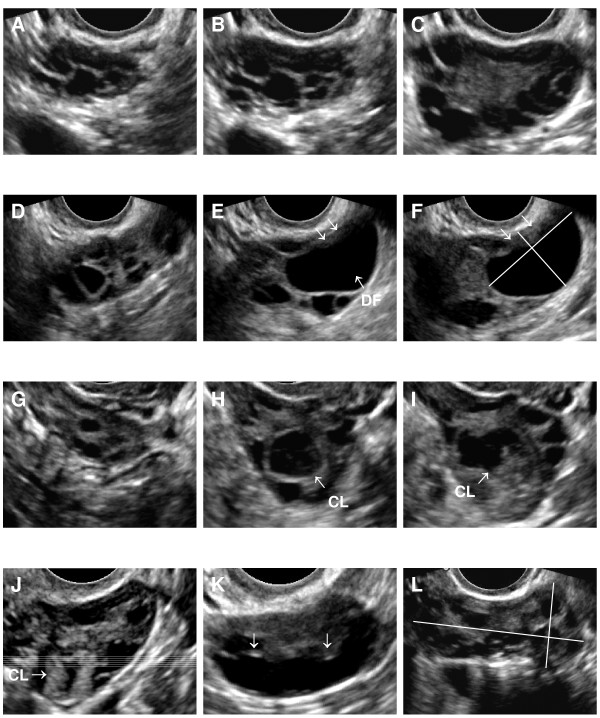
Ultrasonographic image attributes encountered during the inter-observer evaluation of polycystic ovaries are presented. Panels A, B and C represent sequential images through a polycystic ovary where an apparently 'even' distribution of follicles is evident at the end of the ovary while a peripheral distribution of follicles is evident at the central portion of the ovary (Panel C). Designation of follicle pattern was to be made at the largest cross-sectional image of the ovary. Panels D, E and F depict sequential images through a polycystic ovary containing a large dominant follicle (DF). An area of acoustic artifact (arrows) is present along the top portion of the dominant follicle closest to the transducer (Panels E and F). Both the follicle wall and area of acoustic artifact were to be included in the measurements of the largest follicle diameter (Panel F). Panels G, H and I demonstrate sequential images through a polycystic ovary containing a large cystic corpus luteum (CL). The cystic CL has a hyperechoic crenulated border and a flocculent fluid filled cavity. A polycystic ovary containing a CL with thick hyperechoic walls and a small fluid-filled cavity is presented in Panel J for comparison with Panel I. A polycystic ovary containing a concentrated collection of follicles along the lower margin of the ovary is presented in Panel K. The number of follicles counted would depend on the number of lobulations perceived among the "string of pearls". Areas of specular reflection (arrows) could be used to aid in the identification of some individual follicles. A transverse cross-section through an irregular shaped polycystic ovary is shown in Panel L. Estimations of the longest and widest orthogonal diameters of the ovary were to be made for estimations of ovarian volume.
